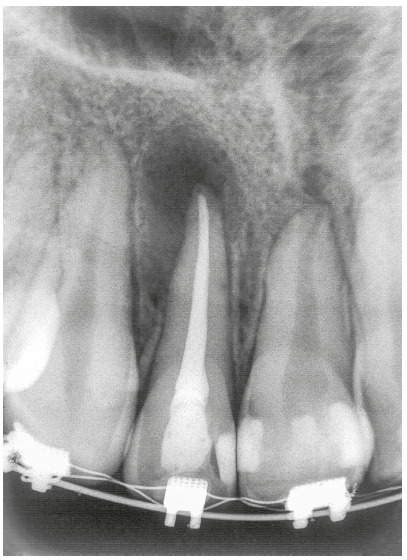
Chronic periapical lesion compatible with immunogenic periapical granuloma treated endodontically in tooth beginning to be moved orthodontically.
